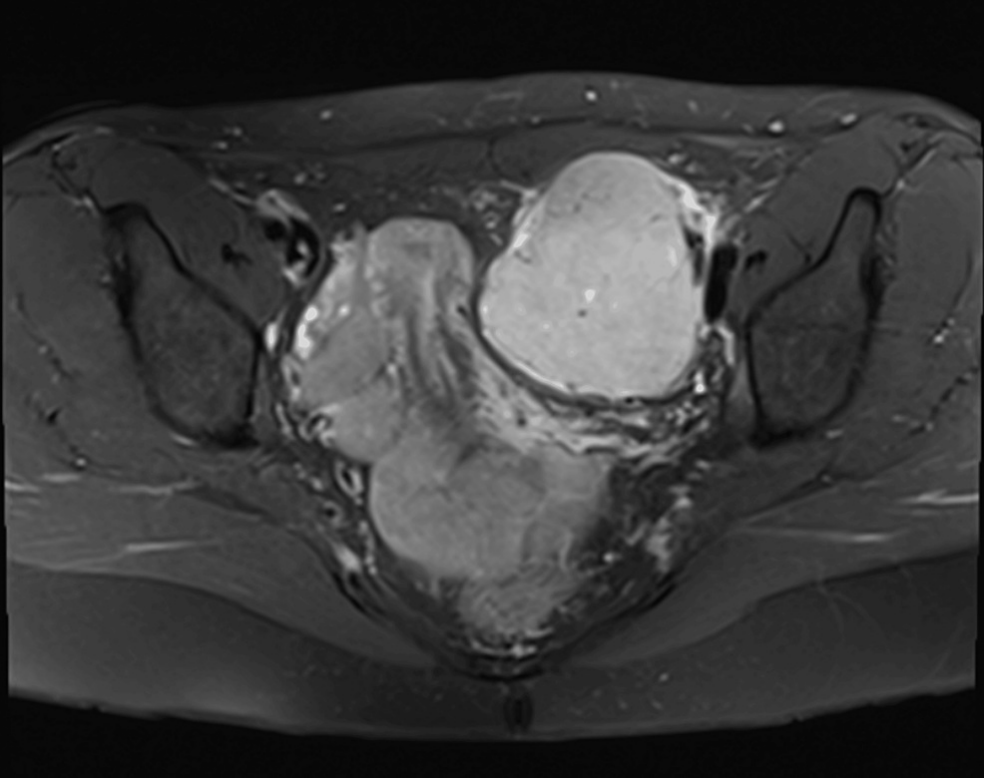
MRI pelvis showing the encapsulated left pelvic sidewall (IDCS) MRI: Magnetic resonance imaging; IDCS: Interdigitating dendritic cell sarcoma
